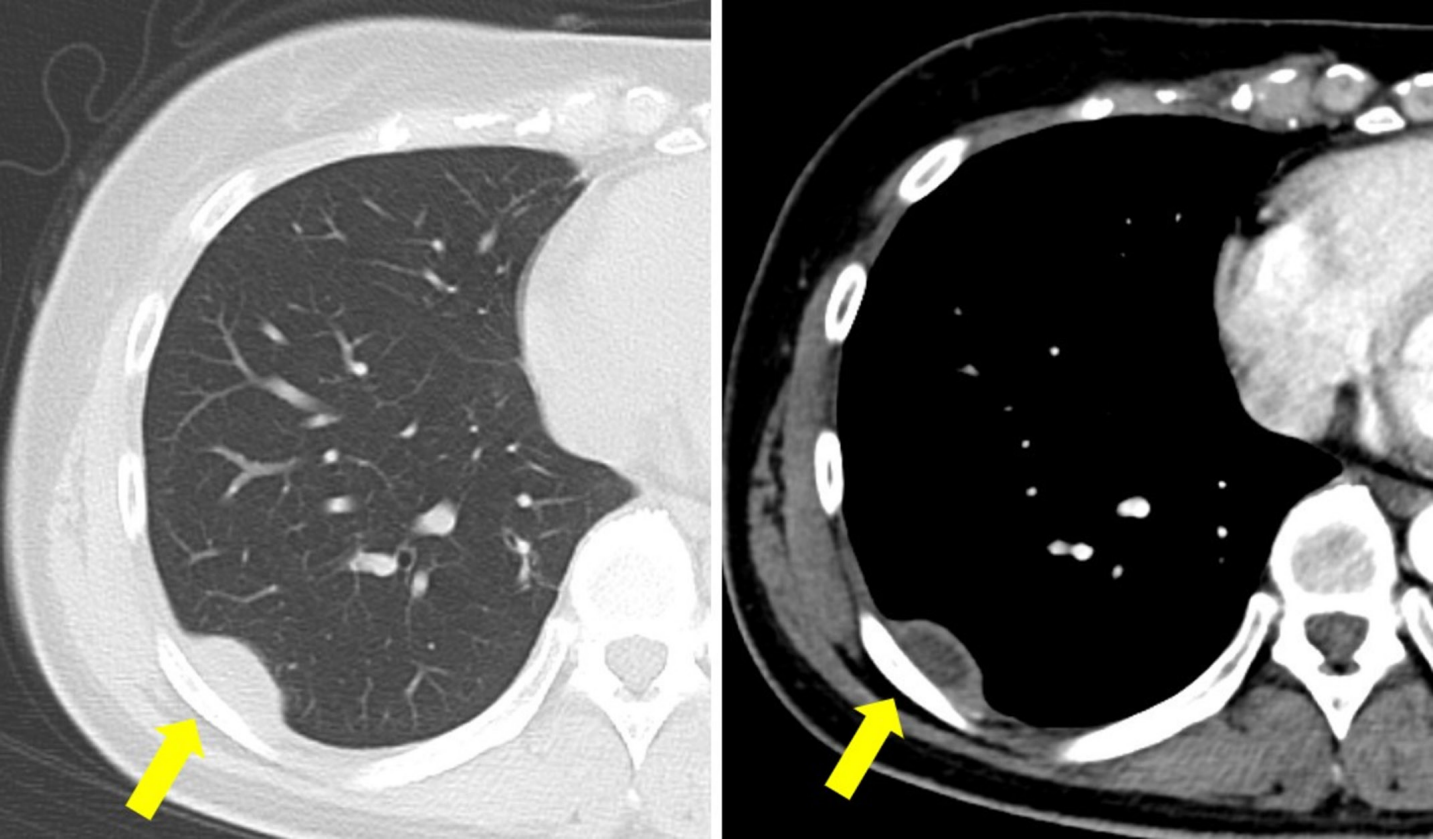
Enhanced computed tomography (CT) scan, demonstrating a tumour moving to the level of number 9th rib. The internal site of the tumour was not enhanced, and the peripheral site of the tumour was enhanced. The tumour was marked with an arrow.
